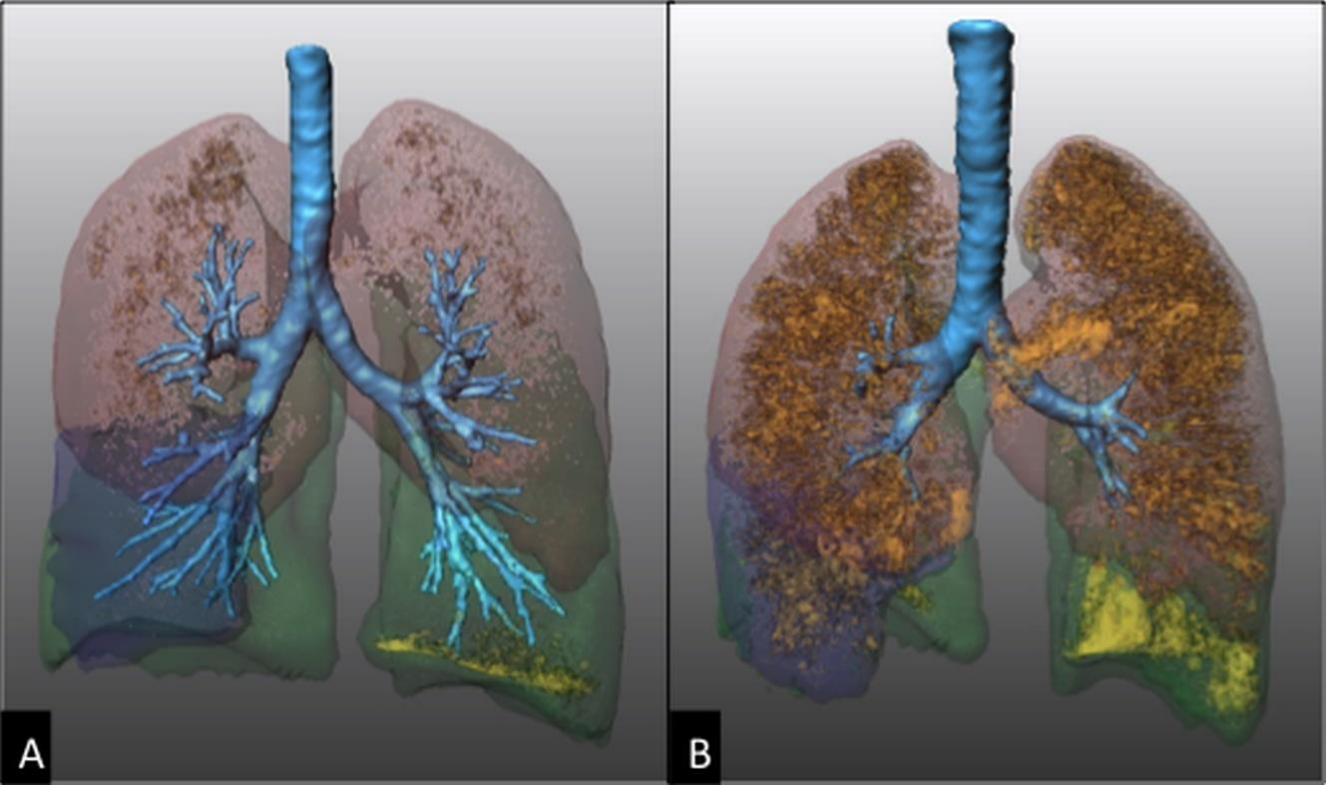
Post-processing imaging techniques: quantification of emphysema and air-trapping Quantification in a 73 years-old woman with COPD by using dedicated software of semi-automatic lung volume segmentation (Franhofer MeVis, Germany, DE). Volume rendering CT images at end-inspiration (A) and end-expiration (B) show pulmonary lobes with different colours and low attenuation areas as orange dots. At end-inspiration (A) the dots represent areas with attenuation values below -950 HU consistent with emphysema, whereas at end-expiration (B) the dots represent areas with attenuation values below -856 HU, consistent with air-trapping and emphysema.
